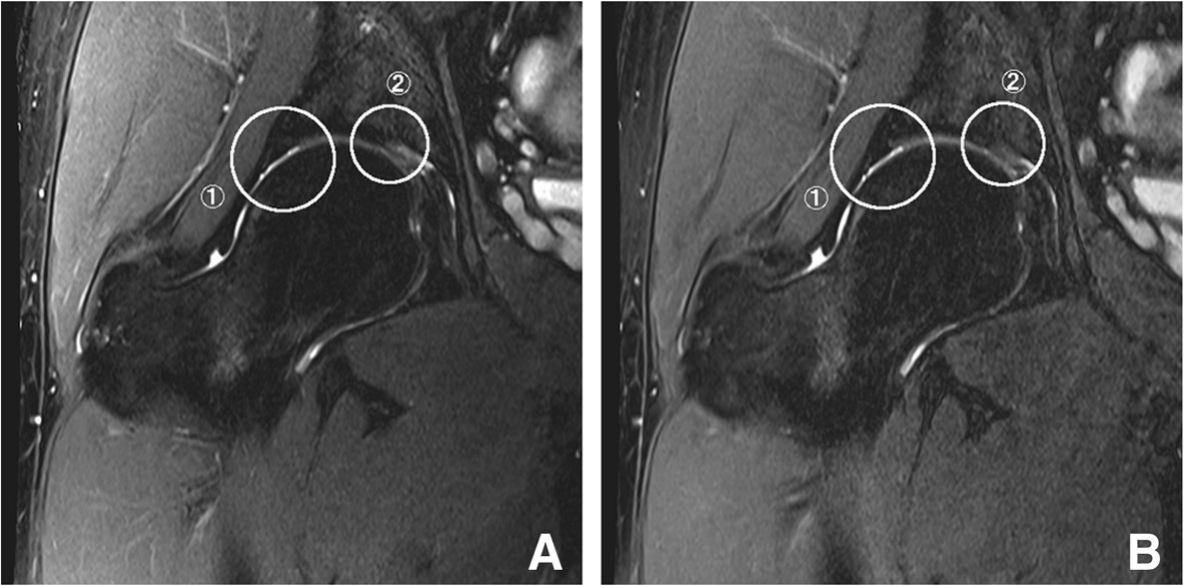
A 22-year-old man with right hip pain. Oblique coronal fat-saturated T2-weighted images with conventional (a) and simultaneous multi-slice sequences (b). Circles indicate areas for image evaluations: ➀ acetabular labrum, ➁ articular cartilage
